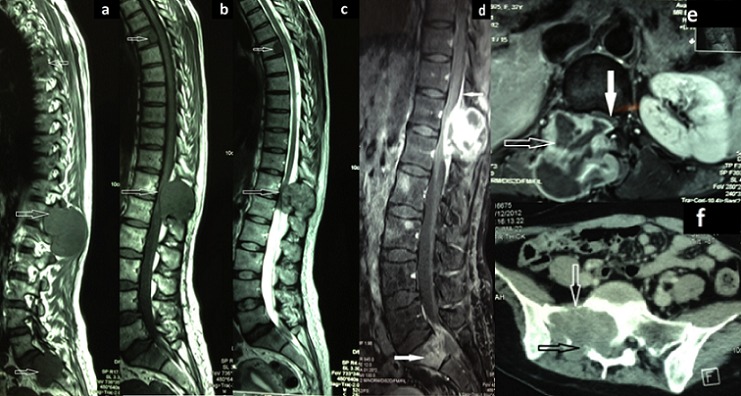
MRI images of the spine in sagittal T1 (a&b), sagital T2 (c) and post contrast sagital (d) and axial (e). The tumor is hypointense to bone on T1 weighted images (a&b), slightly hyperintense and heterogeneous on T2 weighted images (c), with intense, irregular contrast enhancement (d&e). Note the vertebral body involvement in T5 lesion (superior arrows in a, b & c). Note the severe spinal cord compression (middle arrows in a, b, c & e). Note the contrast enhancement along the dura that extends upward and downward (upper arrow in d). Image “f” is an axial CT scan slide showing the sacral lesion with the bone lysis (arrow).
